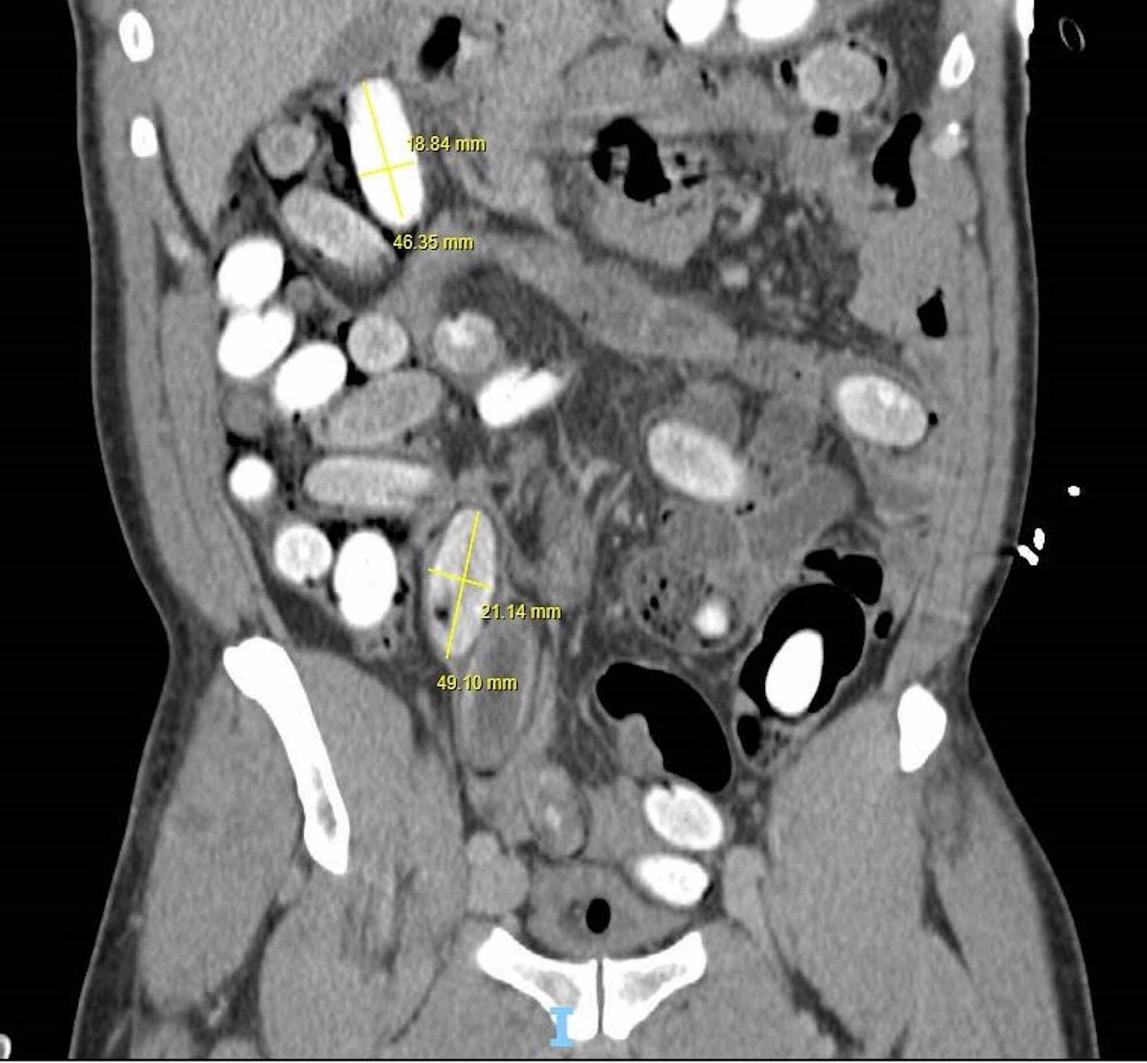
Coronal view of a CT scan showing the cocaine packets distributed in the abdomen.
